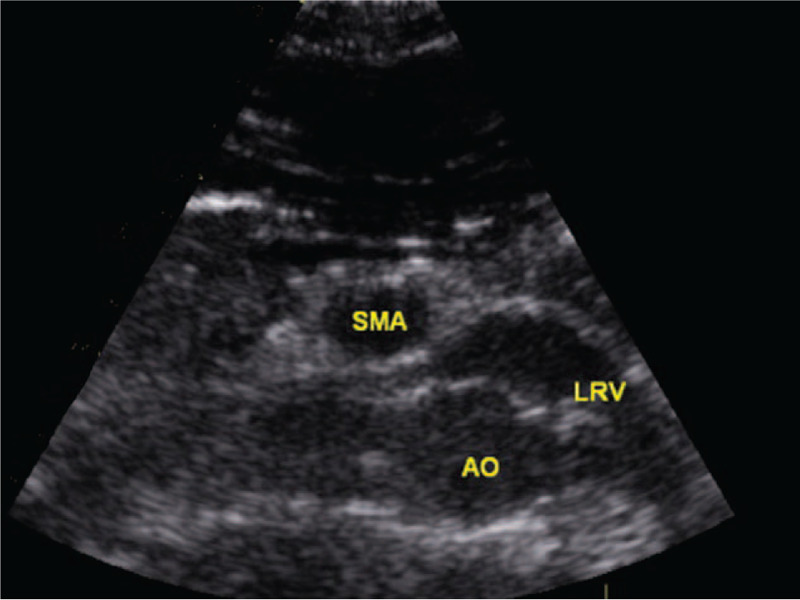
The Doppler ultrasonography revealed that left renal vein (LRV) that runs between the aorta (AO) and the superior mesenteric artery (SMA) is very narrow, which suggested nutcracker phenomenon.
